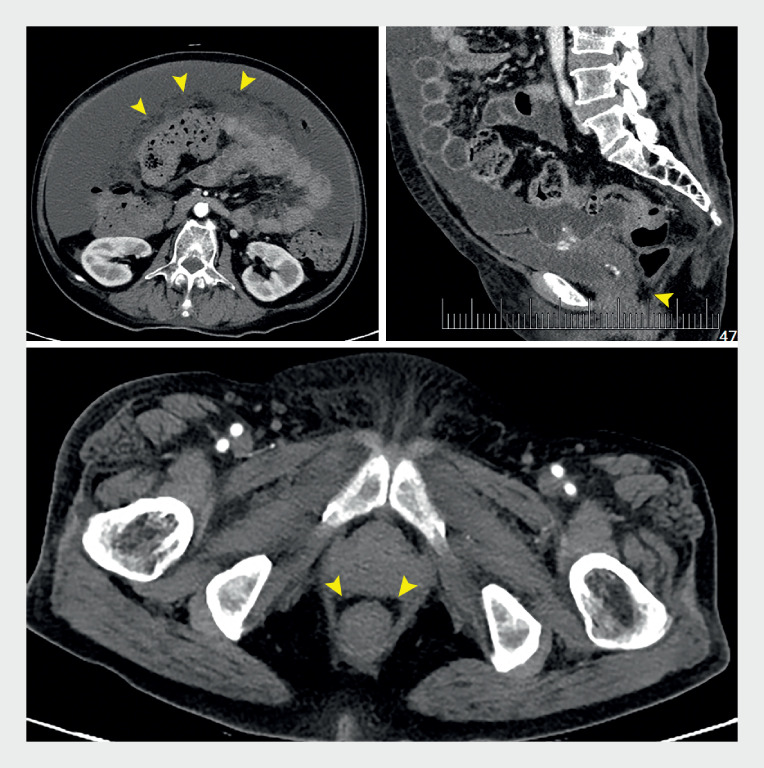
Computed tomography (CT) images; no space-occupying lesions were detected.
